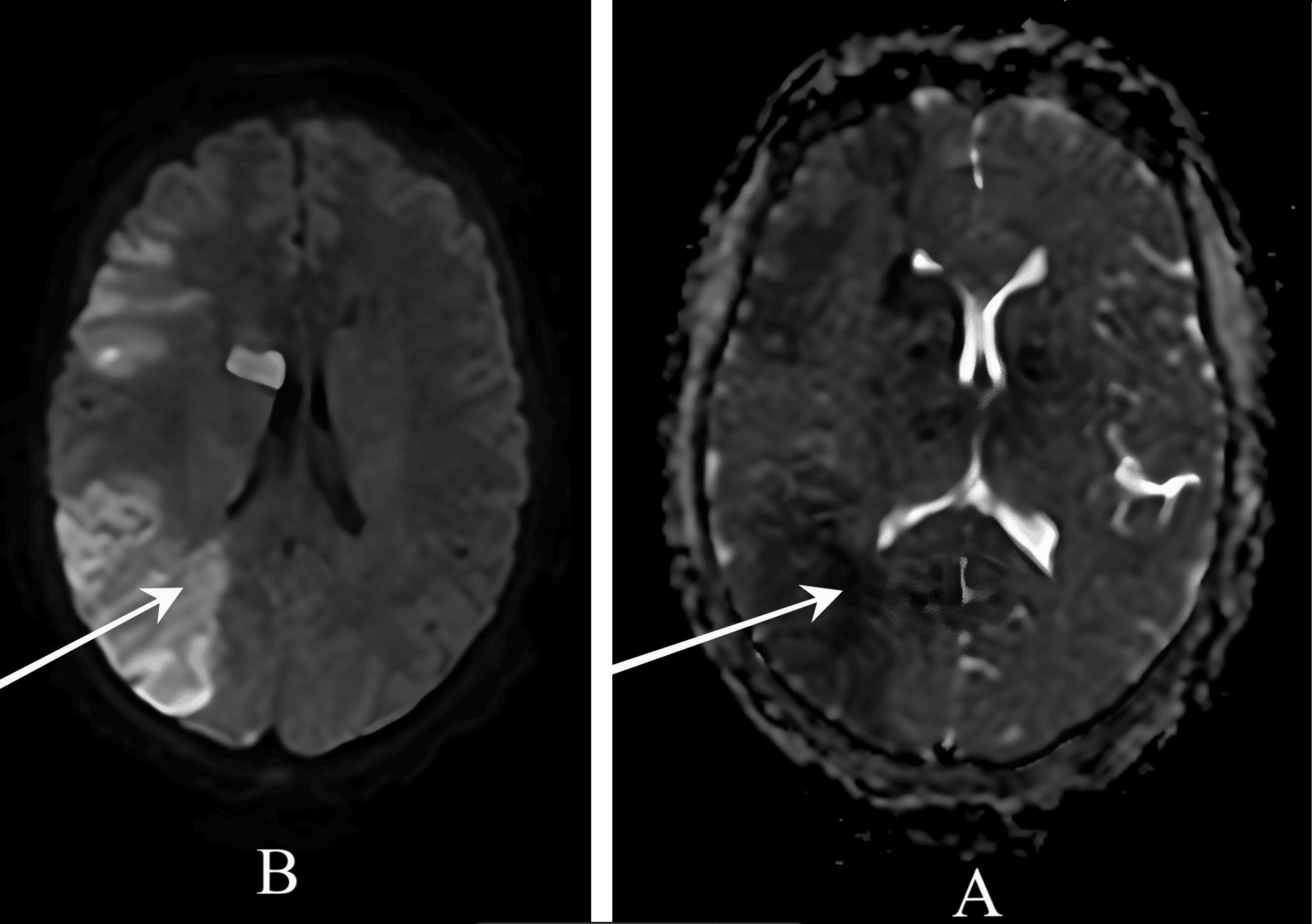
CT Brain demonstrating acute ischemic stroke in the right MCA territory (A) ADC hypo-intensity confirming true diffusion restriction; (B) DWI showing area of restricted diffusion (bright signal) in right MCA territory. MCA: middle cerebral artery; DWI: diffusion-weighted imaging; ADC: apparent diffusion coefficient
